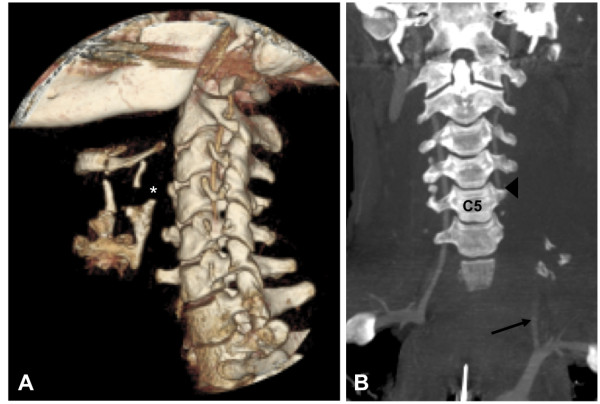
Figure A and B (a) 3D volume rendered (VR) image with fracture of the thyroid cartilage with dislocation of the superior horn on the left side (*). (b) coronal maximum intensity projection of the cervical spine (CT angiography scan after intravenous injection of contrast agent). Proximal abruption of the left vertebral artery (arrow) and retrograde filling (arrowhead) at the level of C5.
